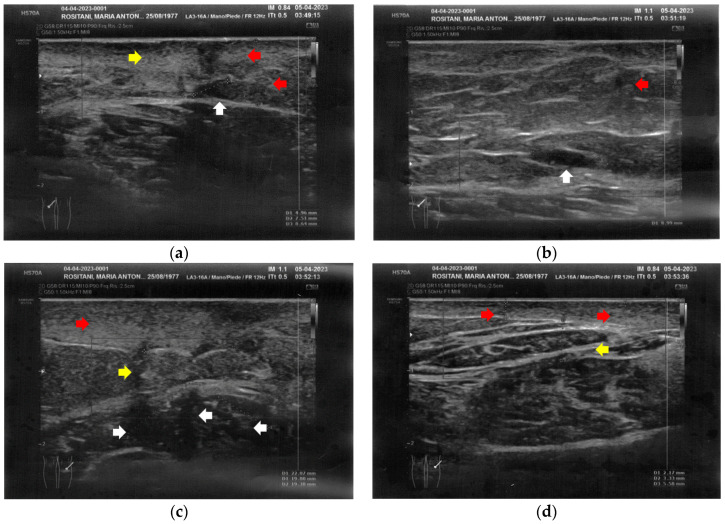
Ultrasonography of the popliteal fossae at T0. (a) Wide section of cicatricial fibrosis (red arrow) of the left popliteal fossa with absence of clear anatomic layers (yellow arrow) and presence of a cicatricial granuloma (white arrow); (b) In another area of the left popliteal fossa the inhomogeneity of the tissues is evident (yellow arrow), and another cicatricial granuloma (white arrow) is present; (c) Cicatricial fibrosis (red arrow) is also evident on the right side, where the adipose tissue appears inhomogeneous (yellow arrows) and where multiple cicatricial granulomas (white arrow) are present in the deeper layer; (d) In another area of the right popliteal fossa, the superficial fibrosis (red arrows) appear less thick, and a partial restructuring of the deeper anatomical layers is noted (yellow arrow).
